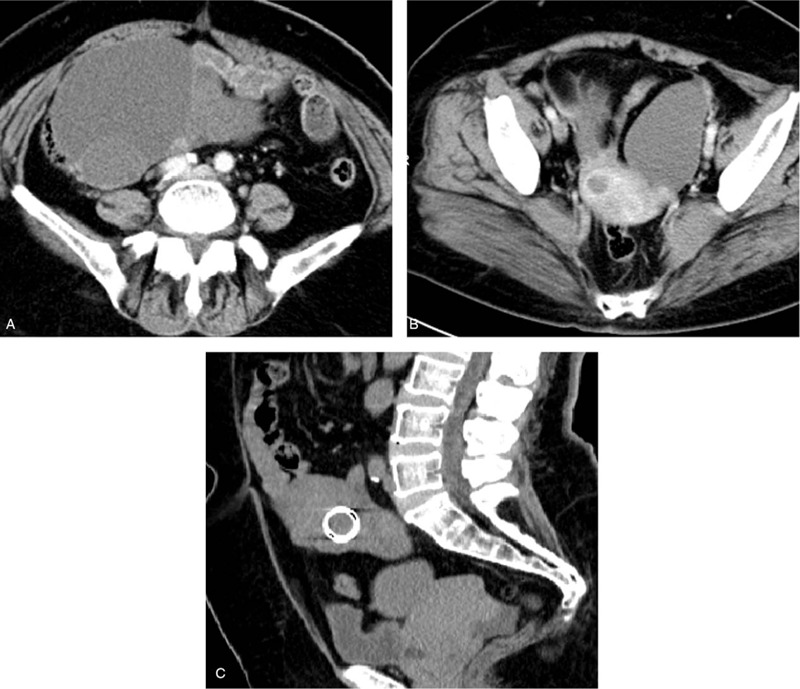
(A) Axial contrast-enhanced CT images showed a cystic mass with enhanced small nodules in the right adnexa. (B) Another cystic mass was also found in the left adnexa. (C) Coronal multiplanar reconstruction confirmed ring hyperdensity within the right ovarian mass, implying migration of the IUD. CT = computed tomography, IUD = intrauterine device.
